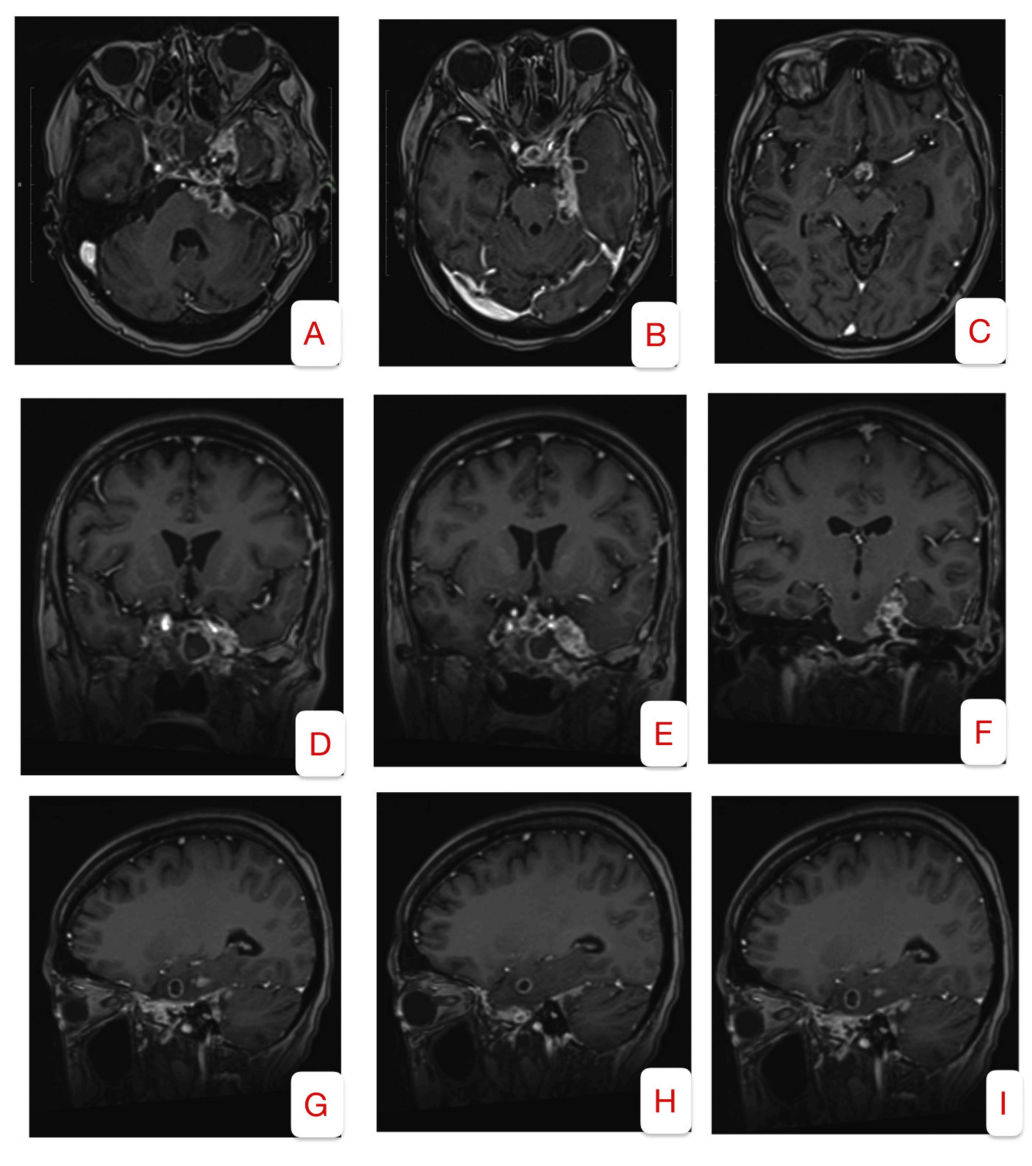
Postoperative contrast MRI A–C (Axial Views): There is a marked reduction in the size of the enhancing mass lesion involving the bilateral cavernous sinuses, the left anterolateral pons, and the left medial temporal lobe. D–F (Coronal Views): Imaging demonstrates a reduction in mass effect on the uncus, with associated improvement in vasogenic edema, as evidenced by decreased cortical effacement. G–I (Sagittal Views): The nasopharyngeal tumor continues to extend to the clivus and prepontine cistern. However, there is a notable reduction in enhancement along the tentorium, and the superior extent of the tumor has significantly decreased.
